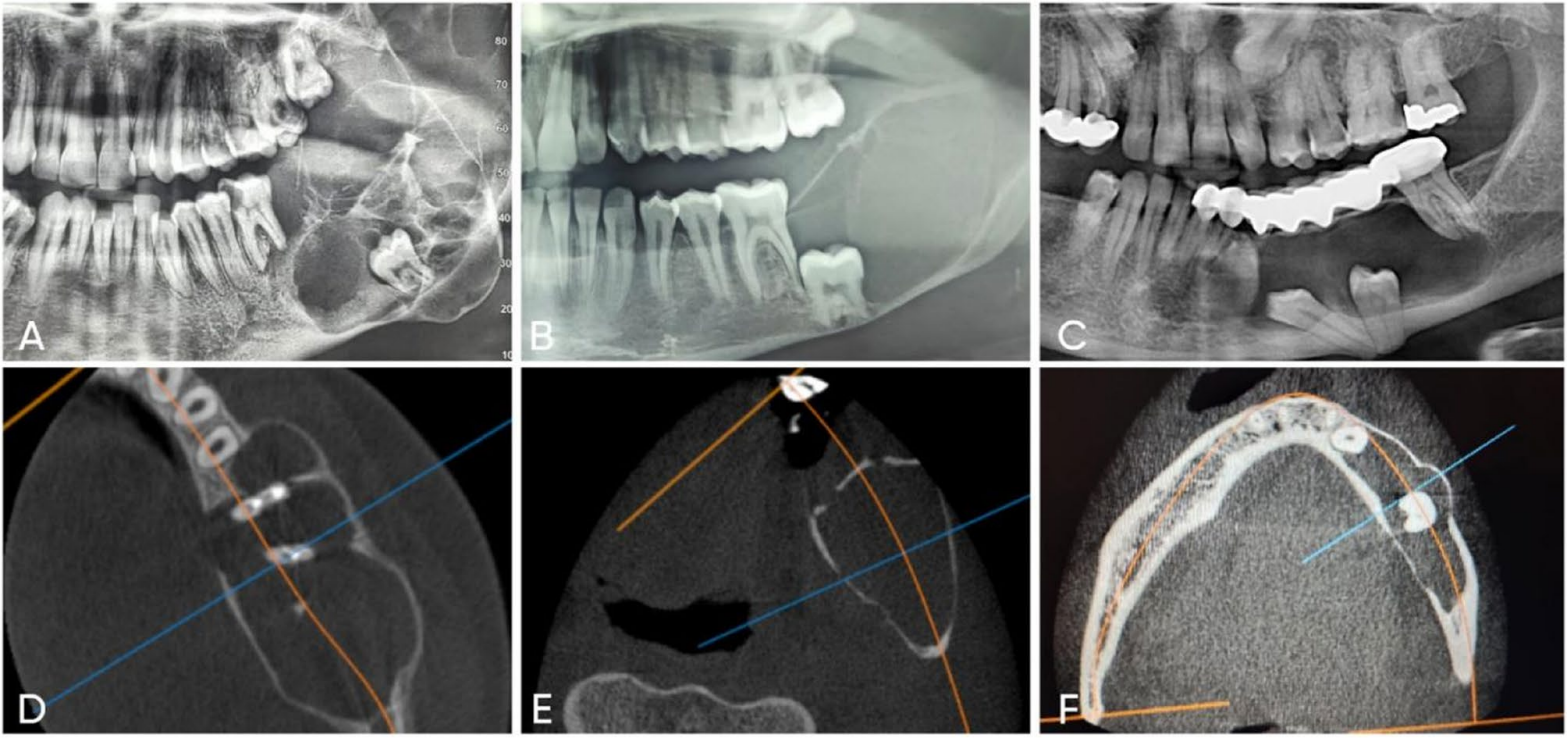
(a) Panoramic x-ray of ameloblastoma shows multilocular radiolucency. b Panoramic x-ray of odontogenic keratocyst shows the unilocular radiolucency. c Panoramic x-ray of dentigerous cyst shows the unilocular radiolucency. d Axial view of CBCT shows lingual bone perforation in ameloblastoma. e Axial view of CBCT shows intact buccal and lingual cortex in Odontogenic keratocyst. f Axial view of CBCT shows intact bone cortex in Dentigerous cyst
